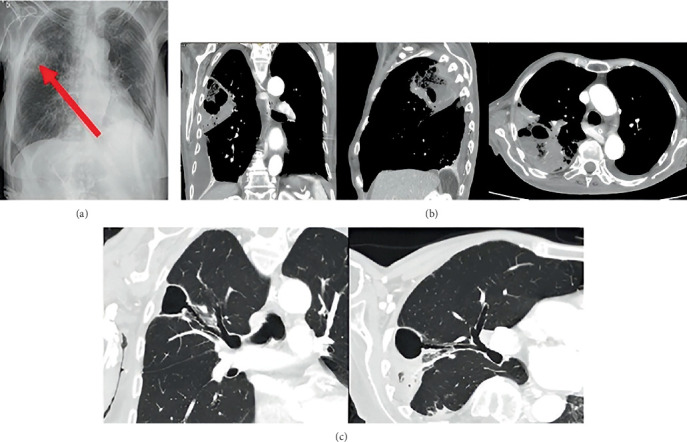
(a) A chest x-ray performed on admission on the 4th of October 2022. CT scans performed on the (b) 7th of October 2022 and (c) 22nd of November 2022—Case 4.
